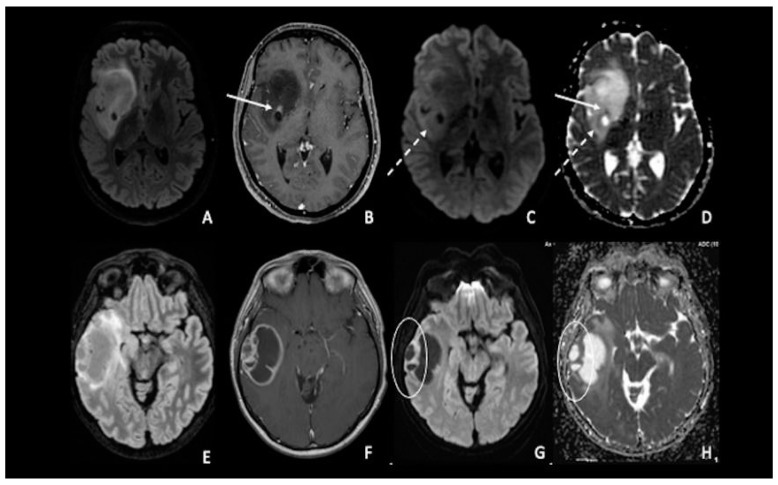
(A–D) IDH-1 mutated glioma in the right Sylvian region. The lesion is characterized by a heterogenous signal on FLAIR (A), with a small area of nodular enhancement on post-contrastographic T1 weighted images (arrow) (B); The site of pathological enhancement was in close proximity to an area of cystic-necrotic degeneration (dotted arrow) (C,D) and showed restricted diffusion on DWI confirmed by the ADC map (arrow); these findings were consistent with an anaplastic behavior of the tumor. (E–H) Right temporal lobe glioblastoma. The lesion was predominantly cystic and had a vivid peripherical post-contrastographic enhancement (F) with a solid component on the lateral side of the tumor; this region presented restricted diffusion on DWI confirmed by the ADC map (circle) (G,H), related to an increase in tumor cellularity. FLAIR = fluid-attenuated inversion recovery; DWI = diffusion-weighted imaging; ADC = apparent diffusion coefficient.
